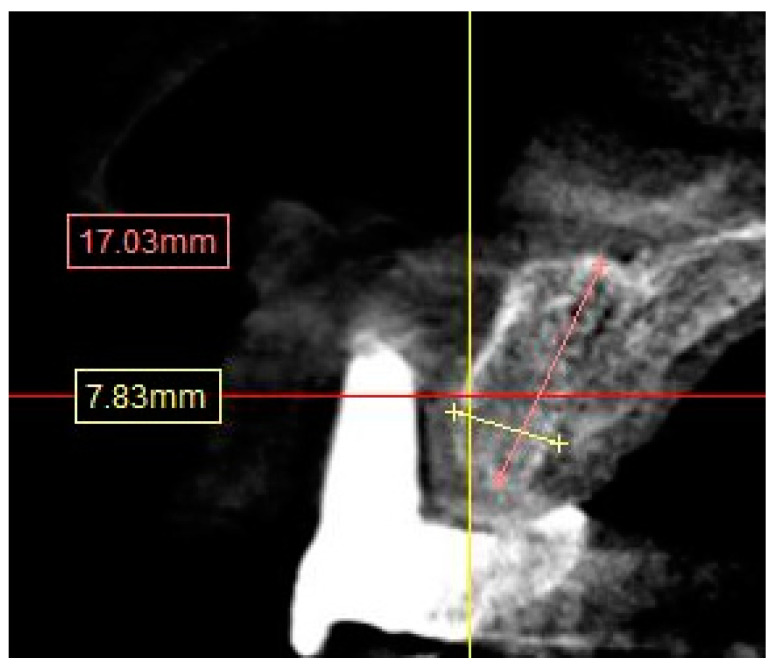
CBCT image showing height and width measurement calculation.
